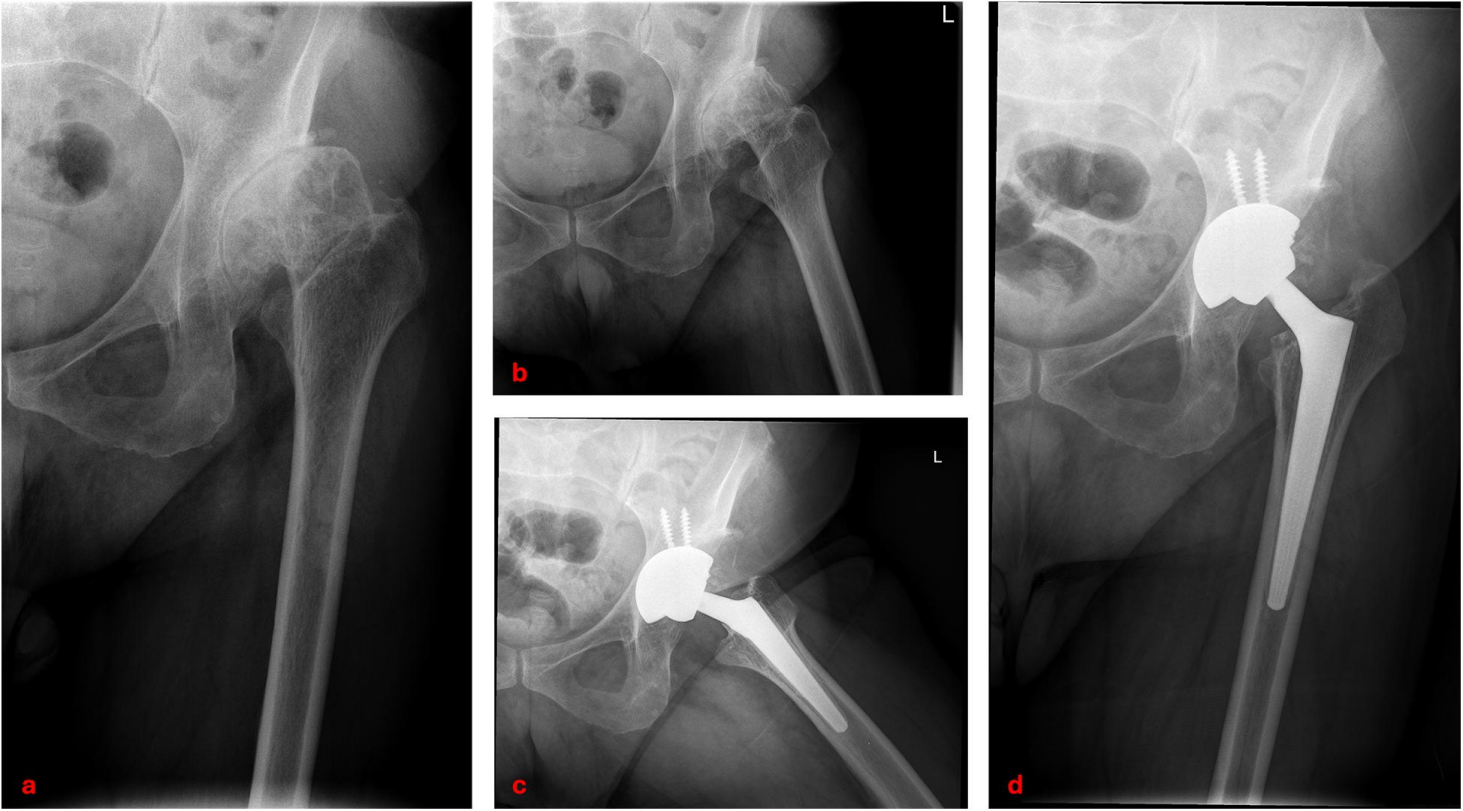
Clinical case of left hip developmental dysplasia (Crowe type III): preoperative anteroposterior and lateral radiographs (a,b); postoperative radiographs at 14-year follow-up showing total hip arthroplasty with Wagner™ conical stem (c,d).
